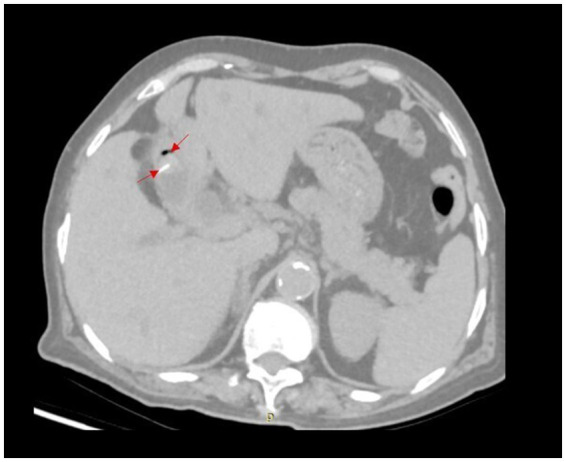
CT imaging reveals gas accumulation in the gallbladder and cord-like foreign bodies.
